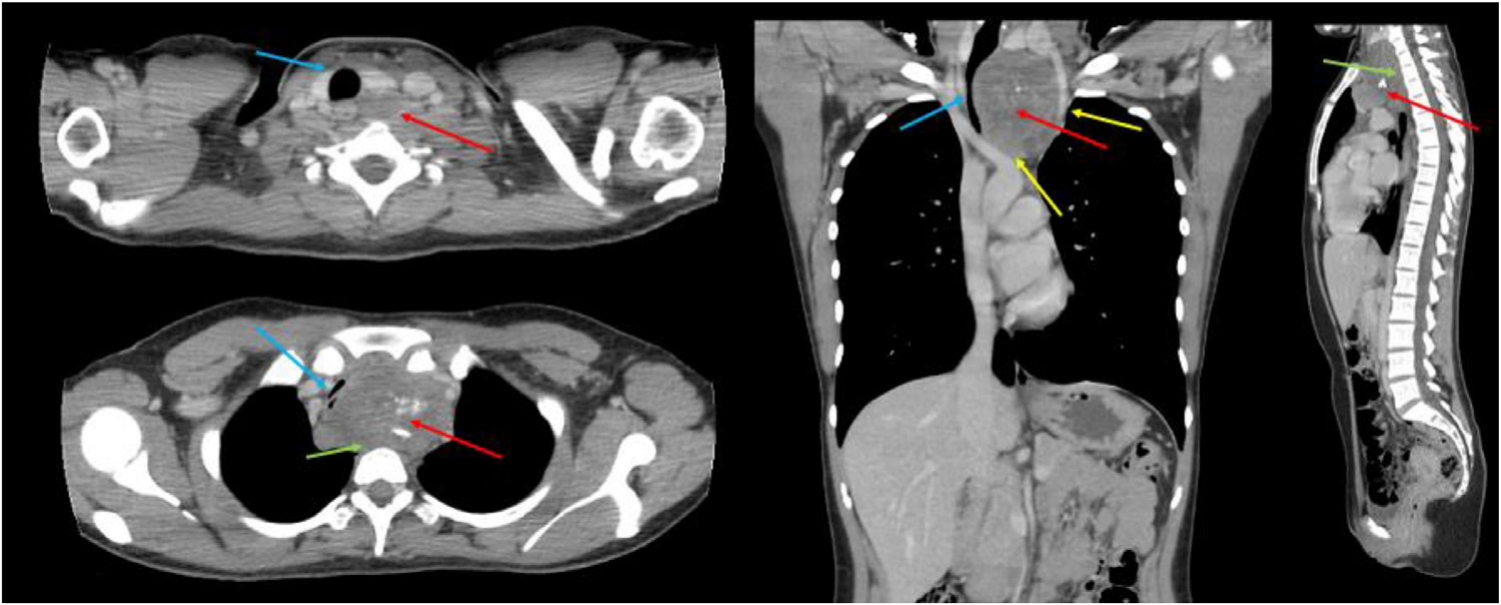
Contrast enhanced axial (top and bottom left), sagittal (right), and coronal (middle) CT images demonstrates heterogenous upper mediastinal mass with internal calcifications (red arrows) that measured approximately 7.5 × 7.4 × 7.6 cm interposed between and splaying the great vessels (yellow arrows). This lesion extends superiorly and inferiorly causing severe left to right mass effect and compression on the trachea (blue arrows). There is abutment of the lesion on the cervicothoracic spine (green arrow). (Color version of figure is available online.)
